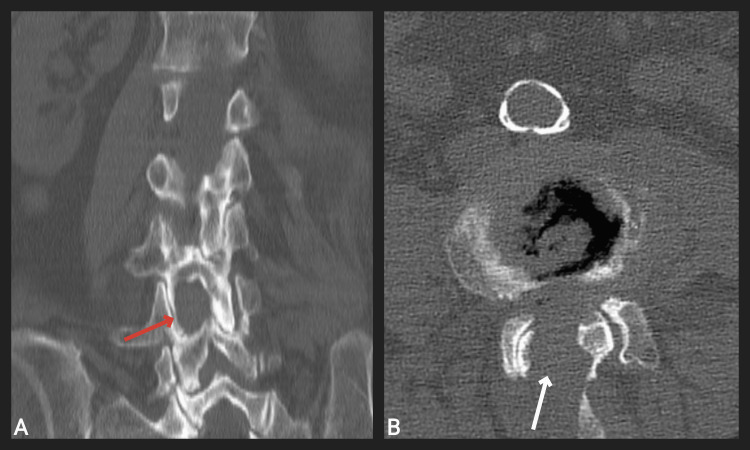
Postoperative CT scan of an 85-year-old woman showing the performed decompression in the coronal plane (red arrow) and axial plane (white arrow) at the L3-L4 level. A: Coronal plane; B: axial plane.
